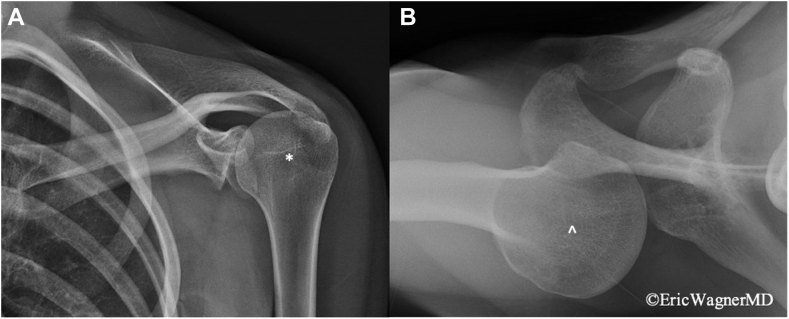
Grashey (A) and axial (B) radiographs of left shoulder. The glenohumeral joint space is poorly visualized on the Grashey view, and the humeral head is superiorly migrated. Axial view demonstrates posterior subluxation of the humeral head, and the glenoid boundaries are challenging to define.
